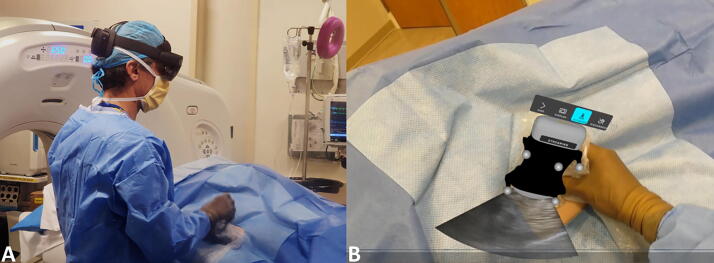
Example of ultrasound image projection along the operative field “through” the patient. (A) External observer perspective. (B) Radiologist perspective through the HMD.
